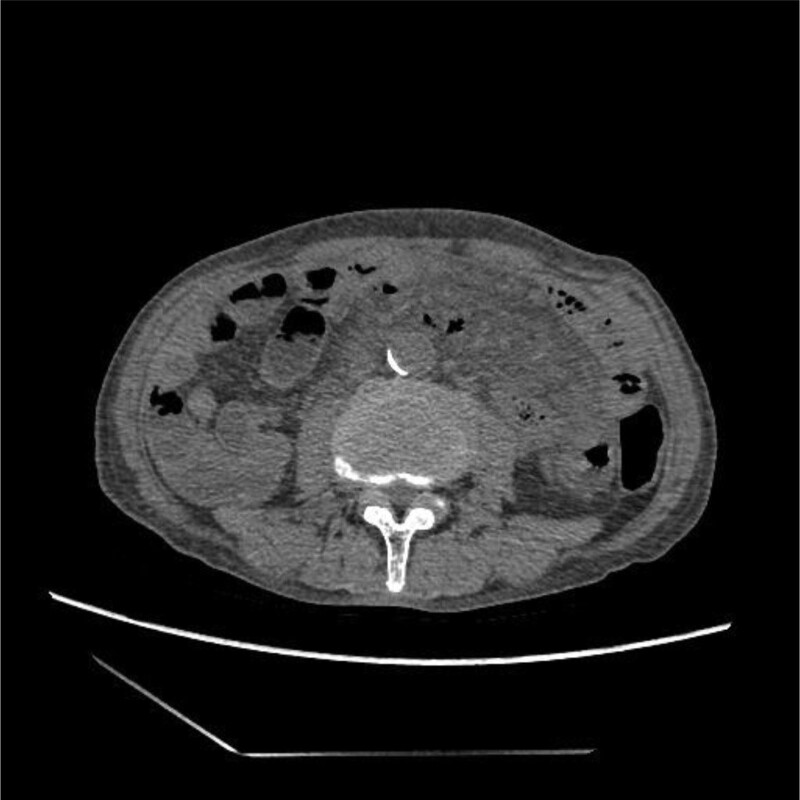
Computerized tomography (CT) showed extensive bubble shadow in mesentery, considering mesenteric abscess, duodenal perforation cannot be excluded.
